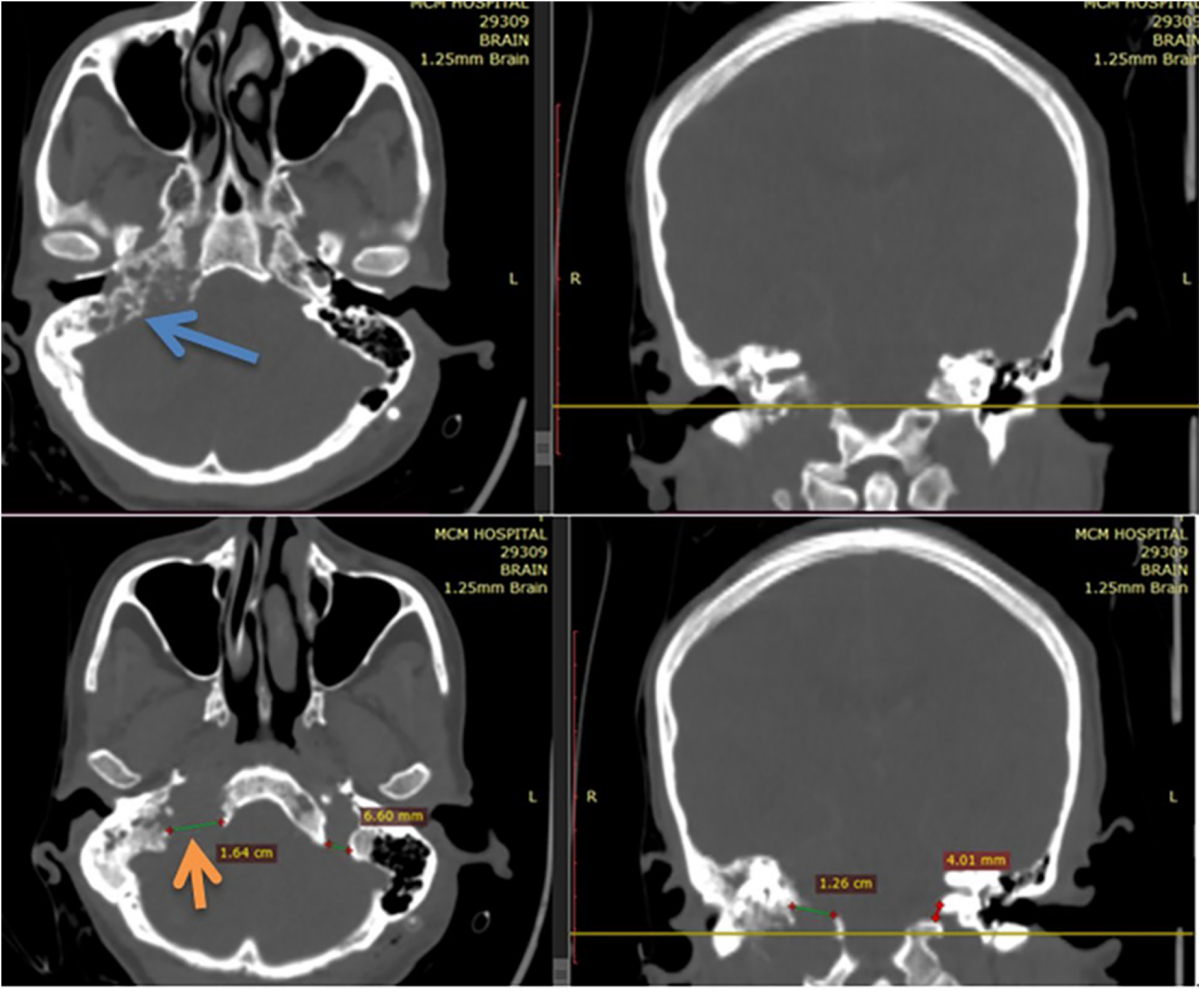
Bone window of CT scan showing an extremely widened right side jugular foramen (depicted by the orange arrow of Figure 4) with a characteristic “moth-eaten” pattern (depicted by blue arrow of Figure 4) of destruction of petrous part of temporal bone.
